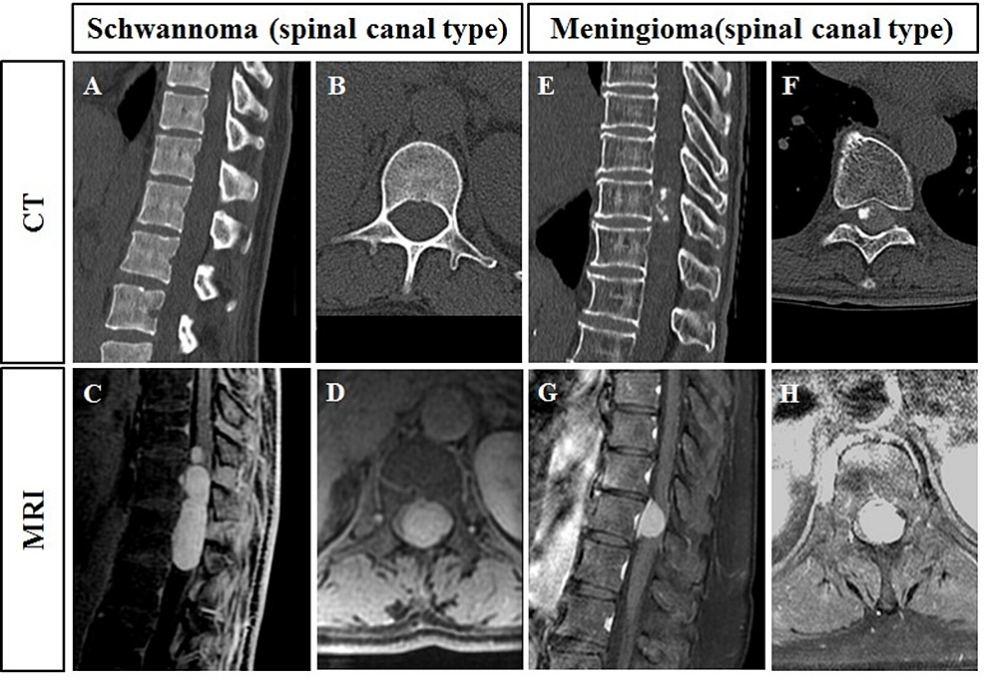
Representative CT and MRI findings of schwannomas and meningiomas. (A–D) Schwannoma (spinal canal type). (E–H) Meningioma (spinal canal type). (A, B, E, F) CT images. (C, D, G, H) Contrast-enhanced MRI findings. CT: computed tomography; MRI: magnetic resonance imaging.
